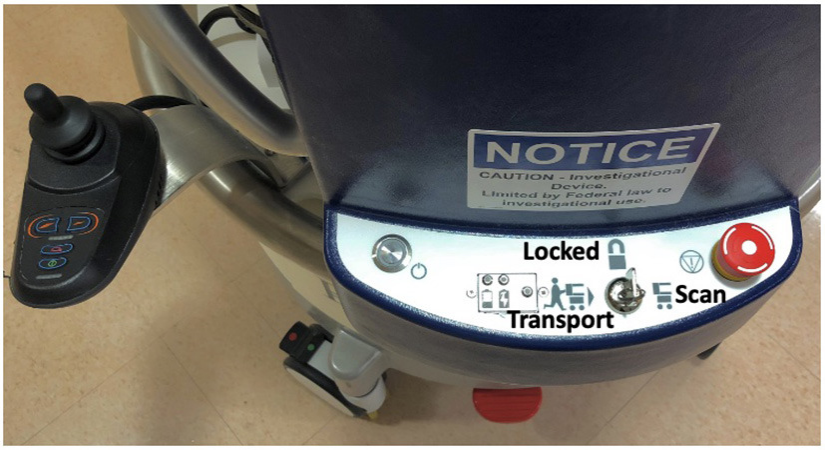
Portable MRI settings. The device has three settings, which can be selected using a key in the control panel. Transport mode (key positioned left) must be selected for the scanner to move, and a joystick on the left side of the control panel is used to steer. There are five speed settings, which can be adjusted by button. Locked mode (key positioned center) is used when the device is stationary and/or charging. Scan mode (key positioned right) is used during scan acquisition.
